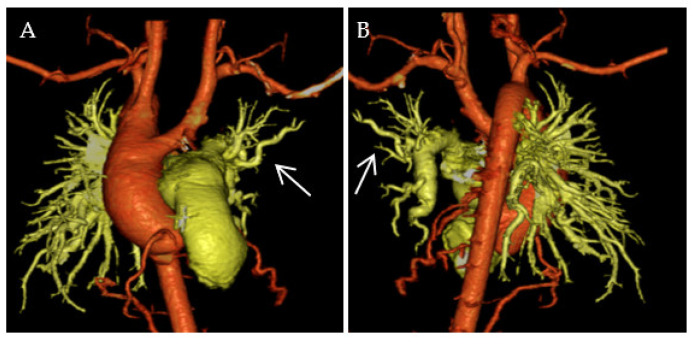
MDCT imaging of repaired TOF and MAPCA in frontal (A) and back (B) views. The left pulmonary arteries are hypoplastic and tortuous (arrows). MAPCA, major aorto-pulmonary collateral arteries; MDCT, multidetector computed tomography; TOF, tetralogy of Fallot.
